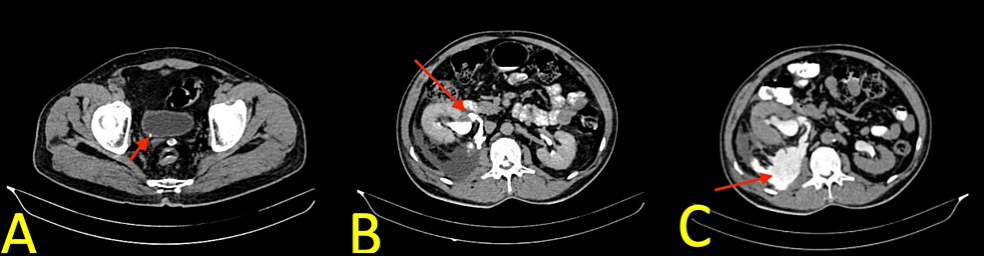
An abdominal computed tomography scan showing (A) a 4 mm calculus at the right vesicoureteral junction (arrow), (B) contrast leak from the renal pelvis (arrow), and (C) urinoma (arrow)
